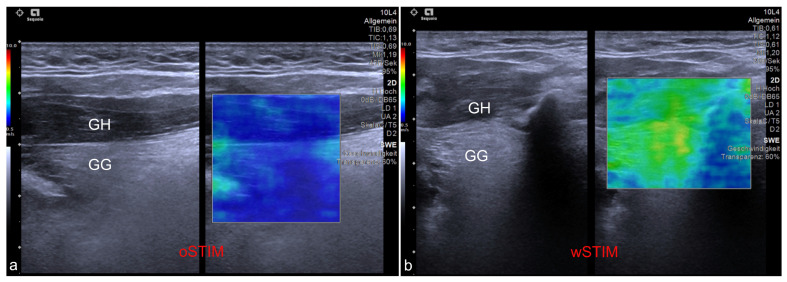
Exemplary ultrasound images showing the application of shear wave elastography at the genioglossus (GG) and geniohyoid (GH) muscles. (a) Measurement of a patient without stimulation of the hypoglossal nerve (oSTIM). (b) Measurement of a patient during stimulation of the hypoglossal nerve (wSTIM).
